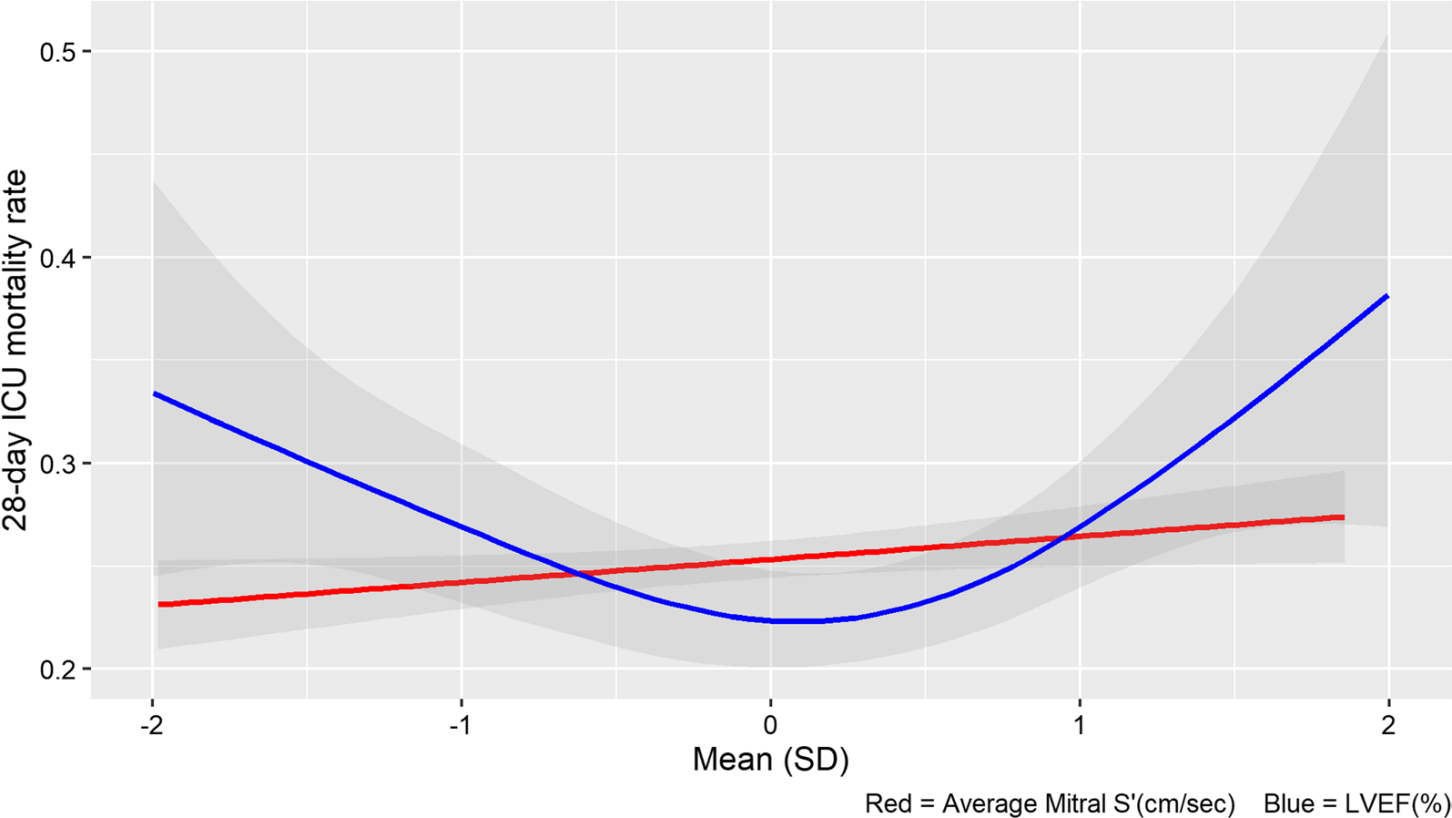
28-day mortality rate and its association with average Tissue Doppler-Derived Left Ventricular Systolic Velocity (mitral S’) and Left ventricle ejection fraction (LVEF %) in patients with sepsis and septic shock
